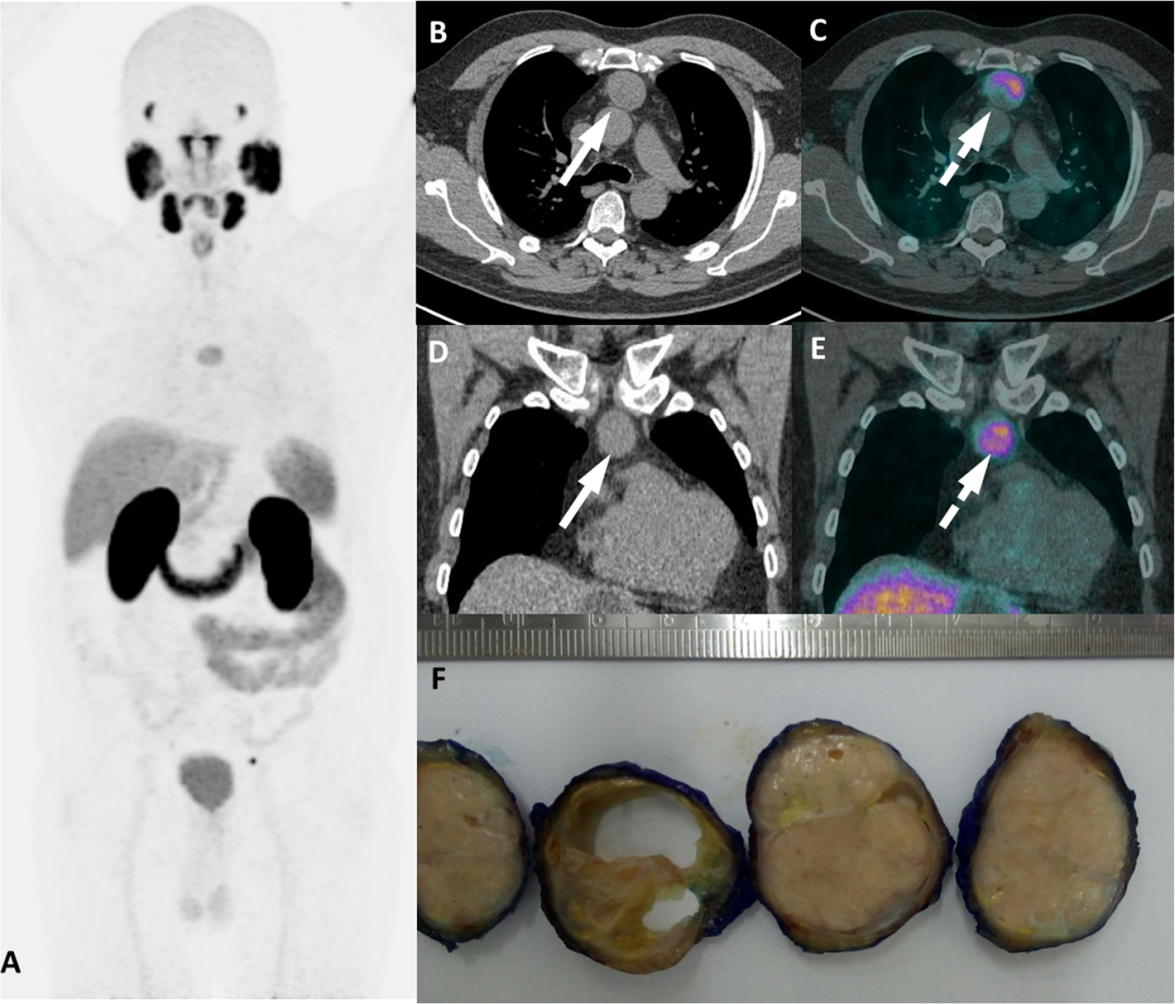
Thymic carcinoma with PSMA uptake. ^68^Ga-PSMA-PET/CT MIP (a), axial and coronal CT (b, d) and axial and coronal PET/CT (c, e) images show an anterior mediastinal mass (arrows) with mild uptake (dashed arrows). As an atypical spread for prostate cancer, this lesion was first biopsied and then resected diagnosed as thymoma as shown in pathology macroscopy (f). *Case courtesy of Bernardo Bacelar, MD and Thiago F. Nunes, MD*
